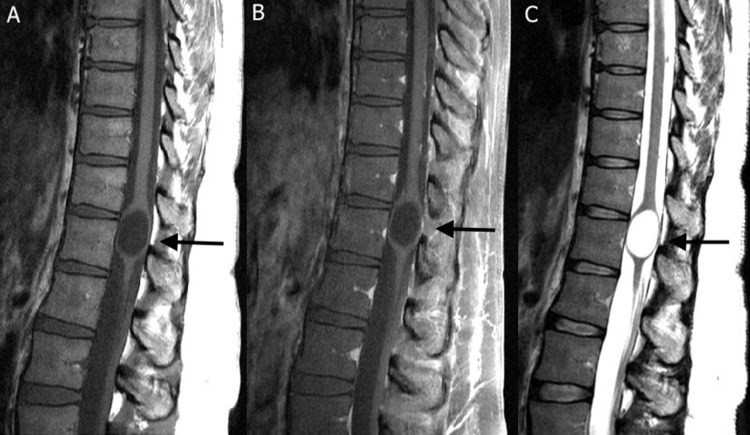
Sagittal MRI thoracic spine demonstrating a cystic lesion near the conus medullaris spanning thoracic level 11 to thoracic level 12. (A) T1 non-contrast sequence; (B) T1 post-contrast; (C) T2.
